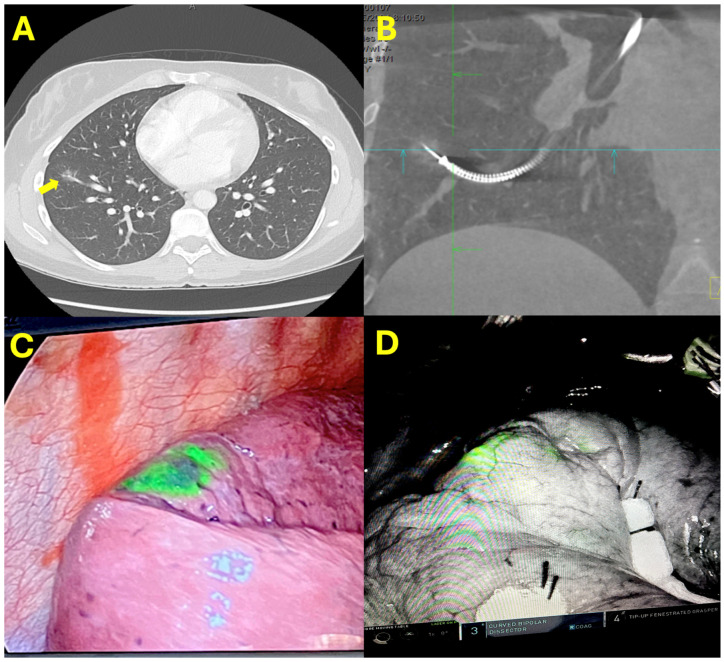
Indocyanine green localization. (A). Right lower lobe ground glass lesion (arrow). (B). Mobile-cone beam CT showing needle in target prior to injection. (C). Indocyanine green view during video-assisted thoracic surgery. (D). Indocyanine green view during robotic-assisted thoracic surgery.
